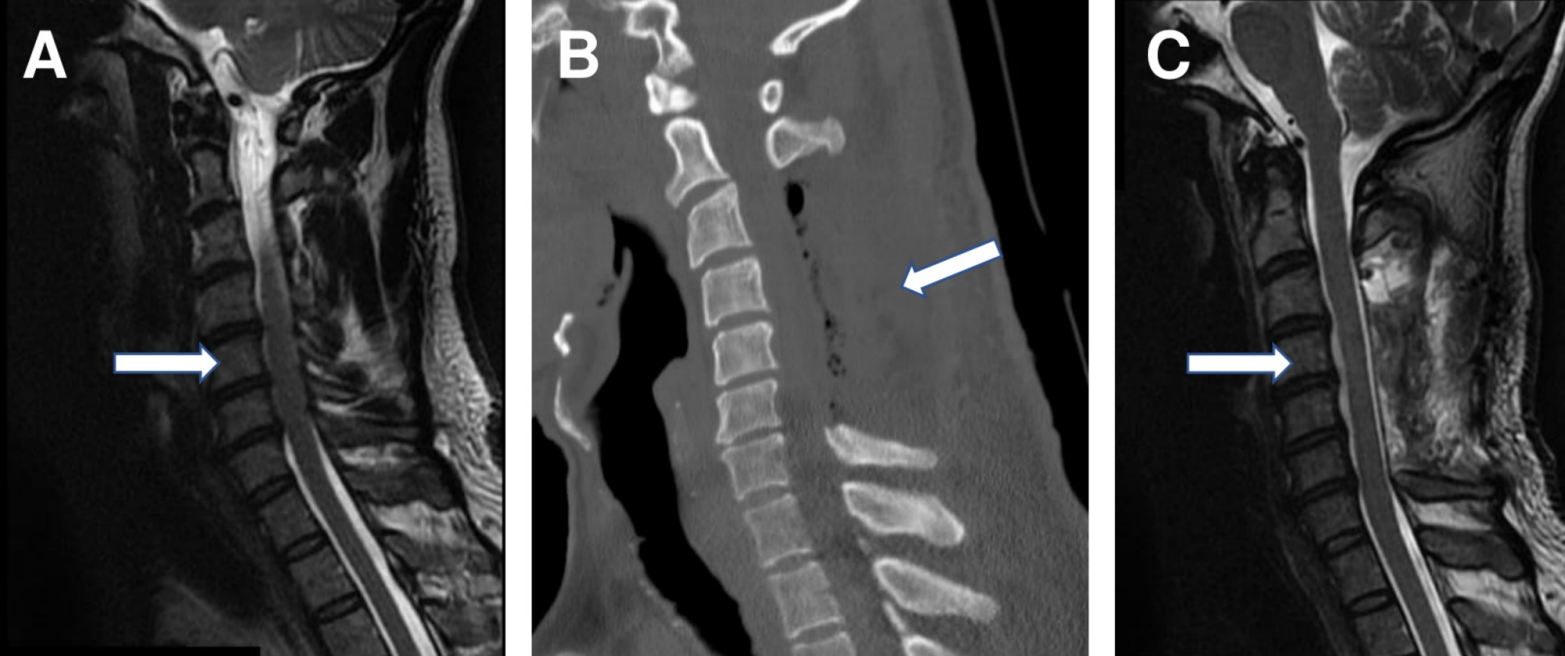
Neutral Position Preoperative and Postoperative Imaging of the Cervical Spine Preoperative sagittal T2 fast recovery fast spin echo magnetic resonance imaging (A) shows cervical spinal stenosis requiring decompression. Postoperative sagittal computed tomography (B) after C3-C6 laminectomy and discectomy. Two weeks postoperative sagittal T2 magnetic resonance imaging (C) shows decompression of cervical spine stenosis.
